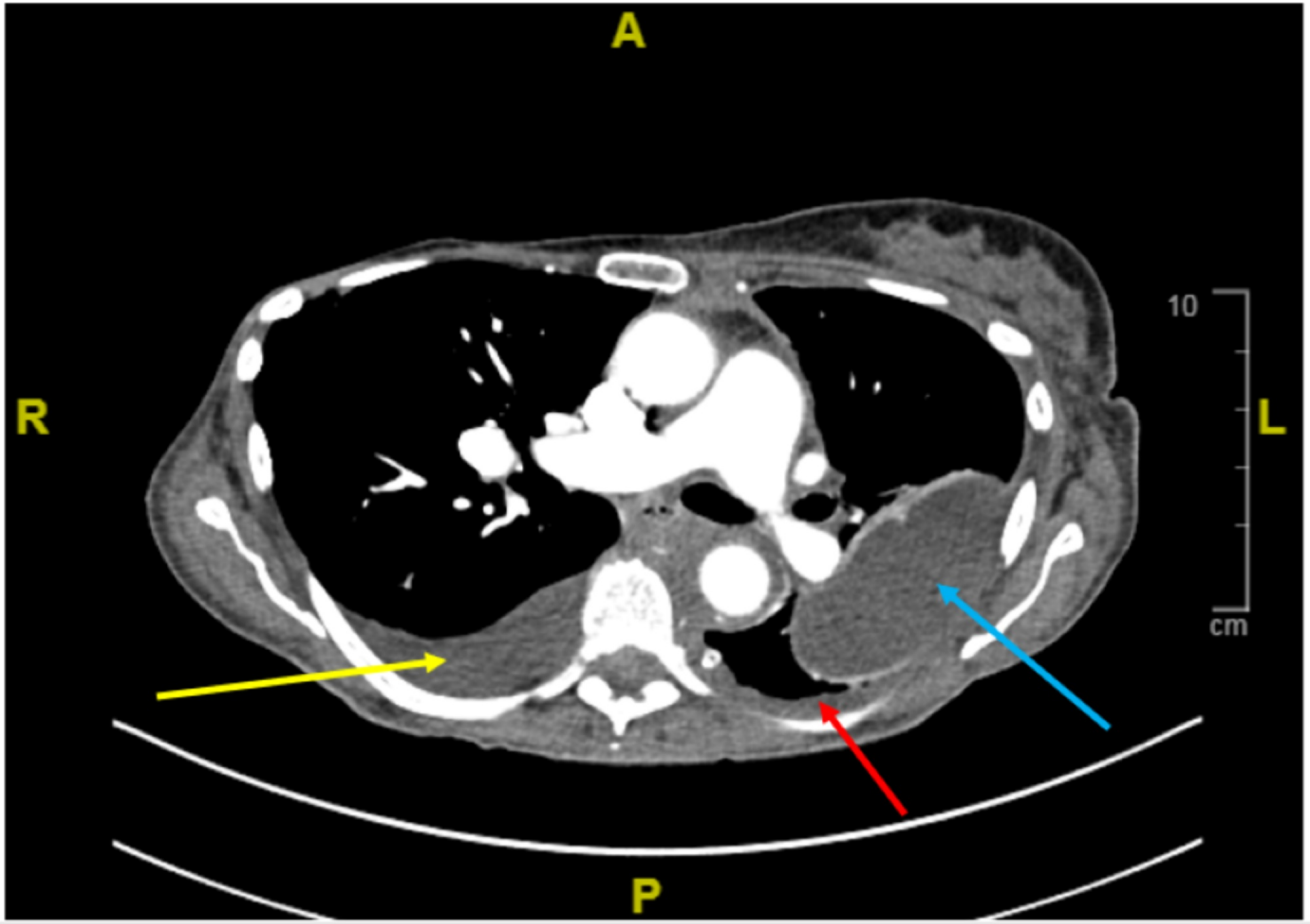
CT pulmonary angiogram. Pulmonary angiogram cross-section at level of pulmonary artery bifurcation. Yellow arrow: There is a moderate right pleural effusion which is increased in extent from prior exam. Blue arrow: There has been an interval decrease in size of the loculated left pleural effusion. Red arrow: There is left-sided pleural thickening and asymmetric soft tissue along the left hemi-diaphragm which demonstrated increased fluorodeoxyglucose (FDG) uptake on recent positron emission tomography (PET) scan examination.
